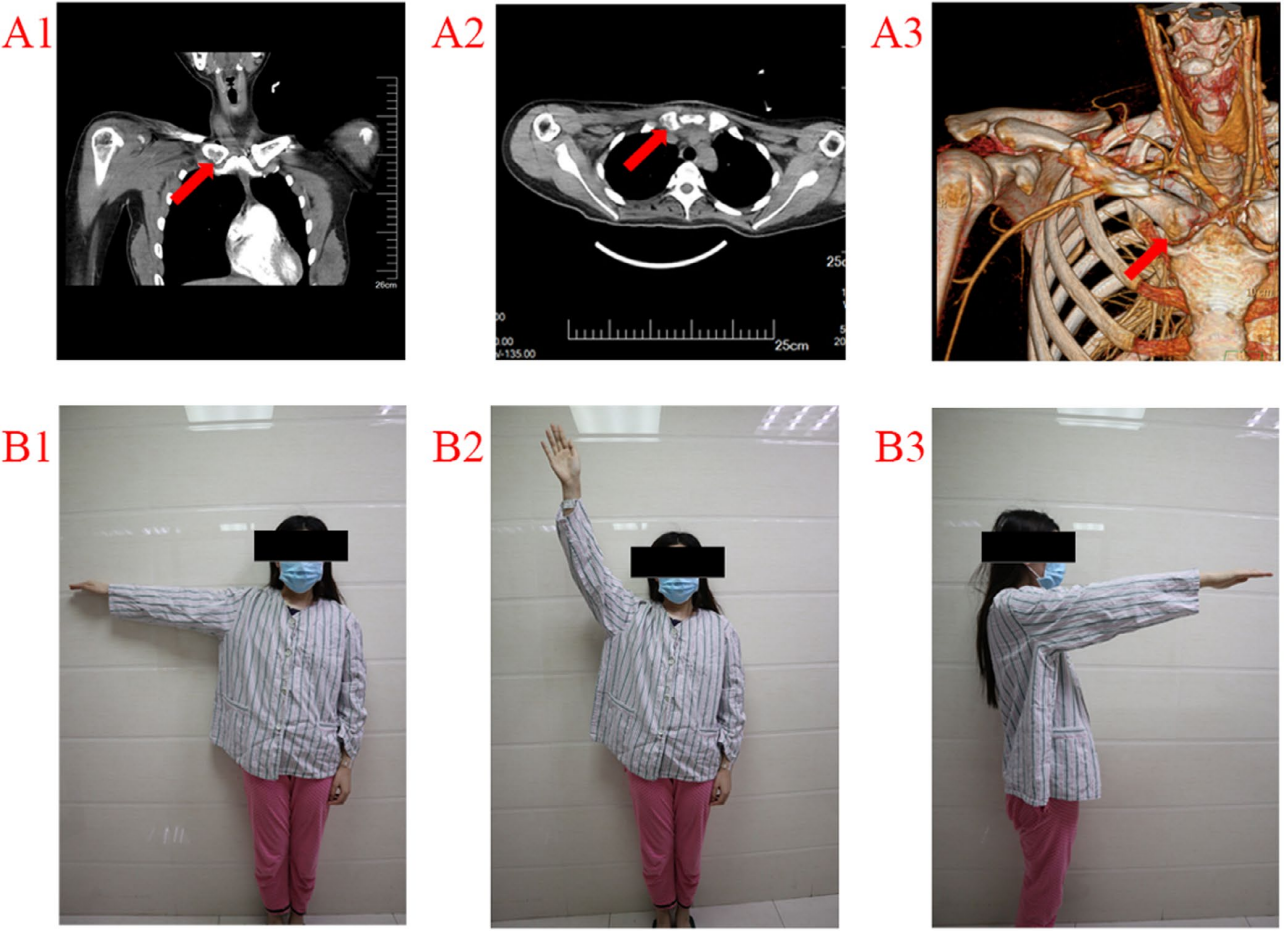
(A1–A3) Postoperative computed tomography scans show the reconstructed sternoclavicular joint. (B1–B3) A satisfactory range of motion of the shoulder after reconstruction of the sternoclavicular joint.
